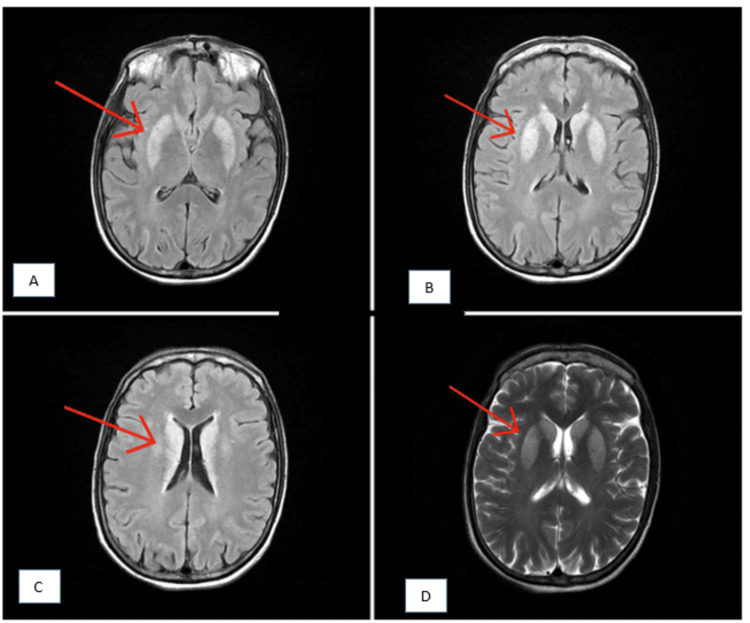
T2-weighted/fluid-attenuated inversion recovery magnetic resonance imaging of the brain showing (A) bilateral symmetrical hyperintensity in the lentiform nucleus, (B) bilateral symmetrical hyperintensity in the corpus striatum, (C) periventricular white matter hyperintensity, and (D) bilateral symmetrical hyperintensity in the corpus striatum.
